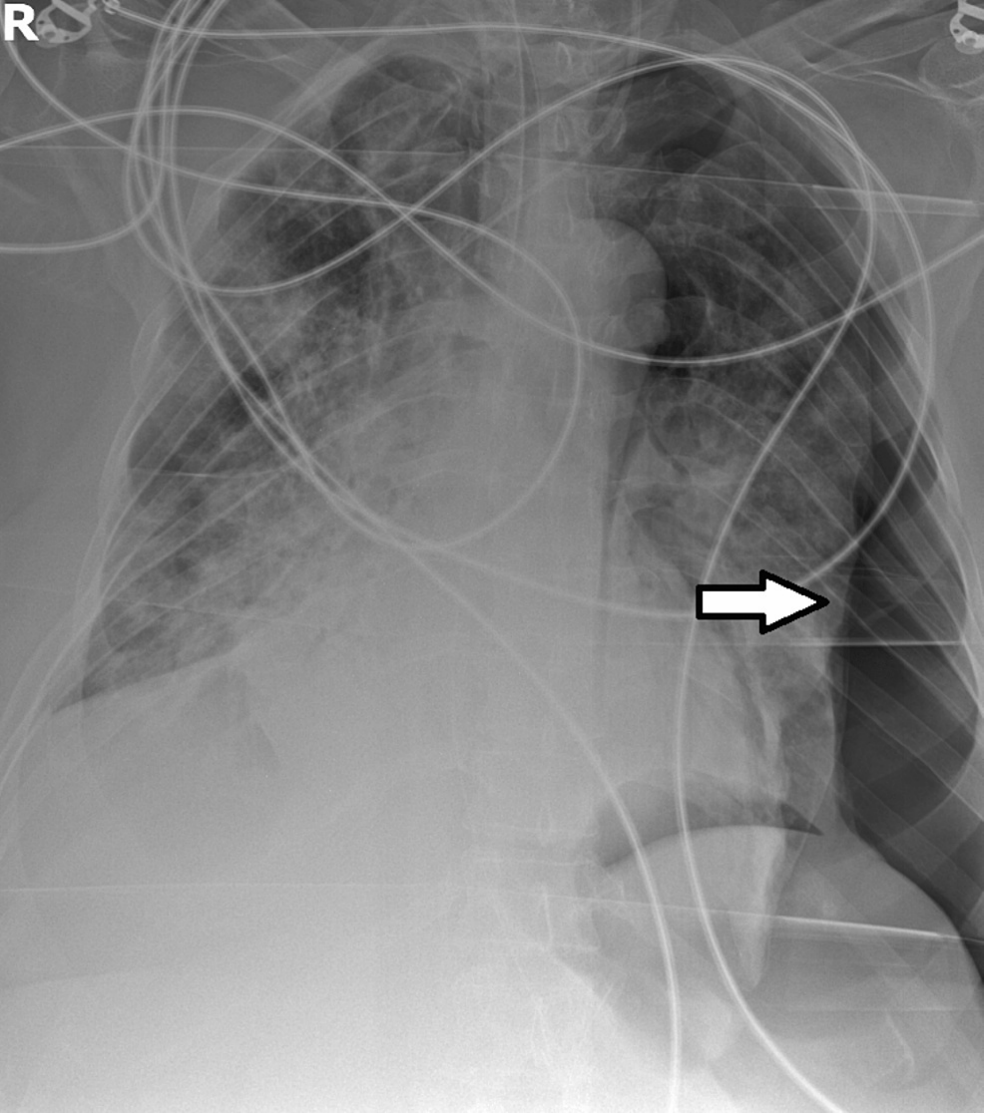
Radiograph of the thorax on the 10th day in the intensive care unit Left pneumothorax (arrow) is evident.
